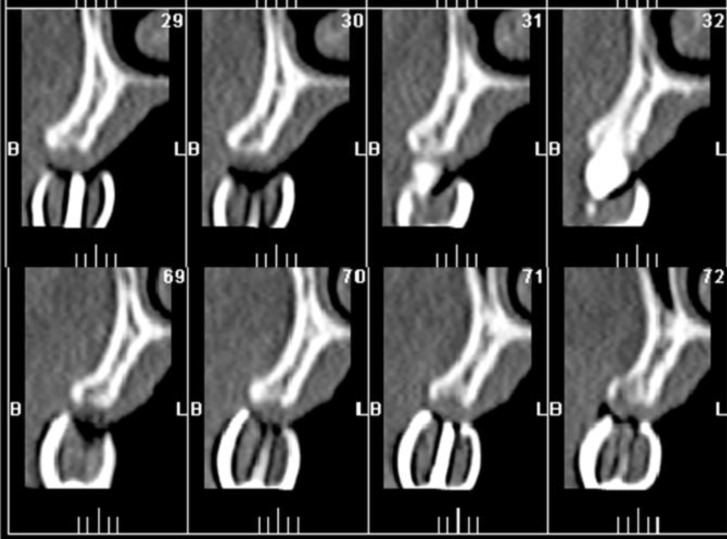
CT evaluation with radiographic stent
